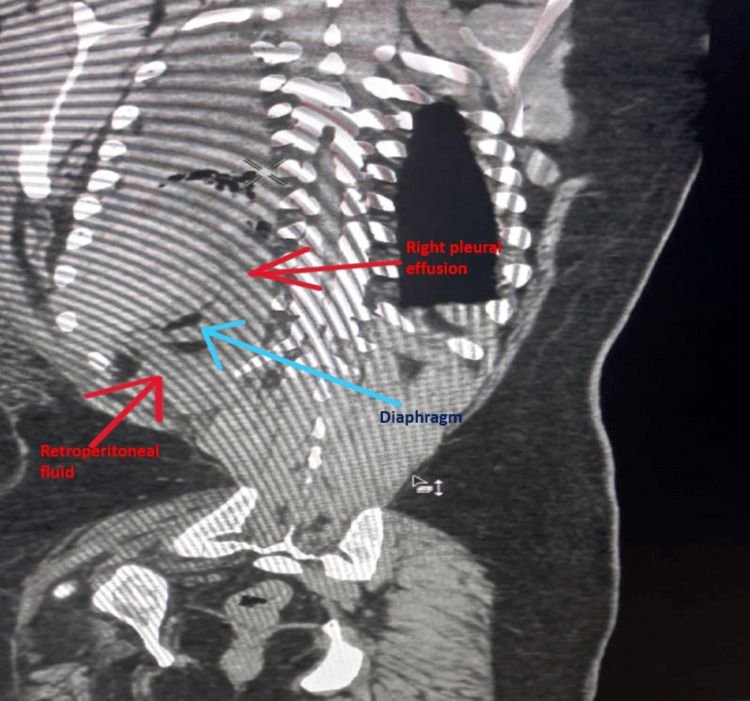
Coronal CT reconstruction demonstrating cranial extension of retroperitoneal fluid into the right pleural cavity Coronal noncontrast CT reconstruction demonstrating retroperitoneal fluid (lower arrow) extending cranially along retroperitoneal fascial planes toward and through the right hemidiaphragm, with associated right-sided pleural effusion (upper arrow). This imaging pattern supports suspected communication between the retroperitoneal space and the pleural cavity, consistent with urinothorax. The proposed mechanism includes either direct passage through a diaphragmatic defect or indirect migration via lymphatic channels under elevated retroperitoneal pressure CT: computed tomography
